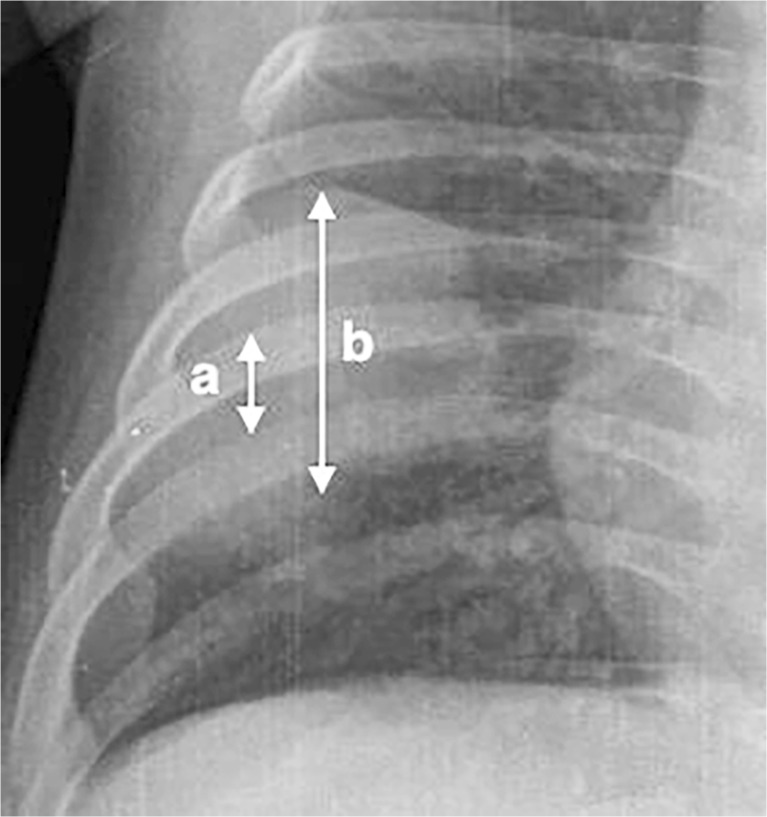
Anteroposterior radiograph cropped to the right hemithorax of a 2 month old male hospitalized with WHO-defined very severe clinical pneumonia and meningitis. Reference measurement of one posterior rib and the adjacent rib space (*double-arrow*
